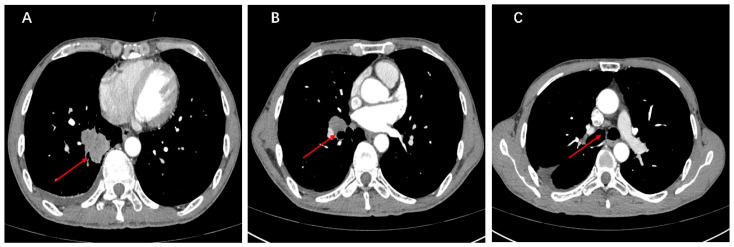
(A–C) Preoperative CT of the chest revealed a space-occupying lesion in the lower lobe of the right lung.
